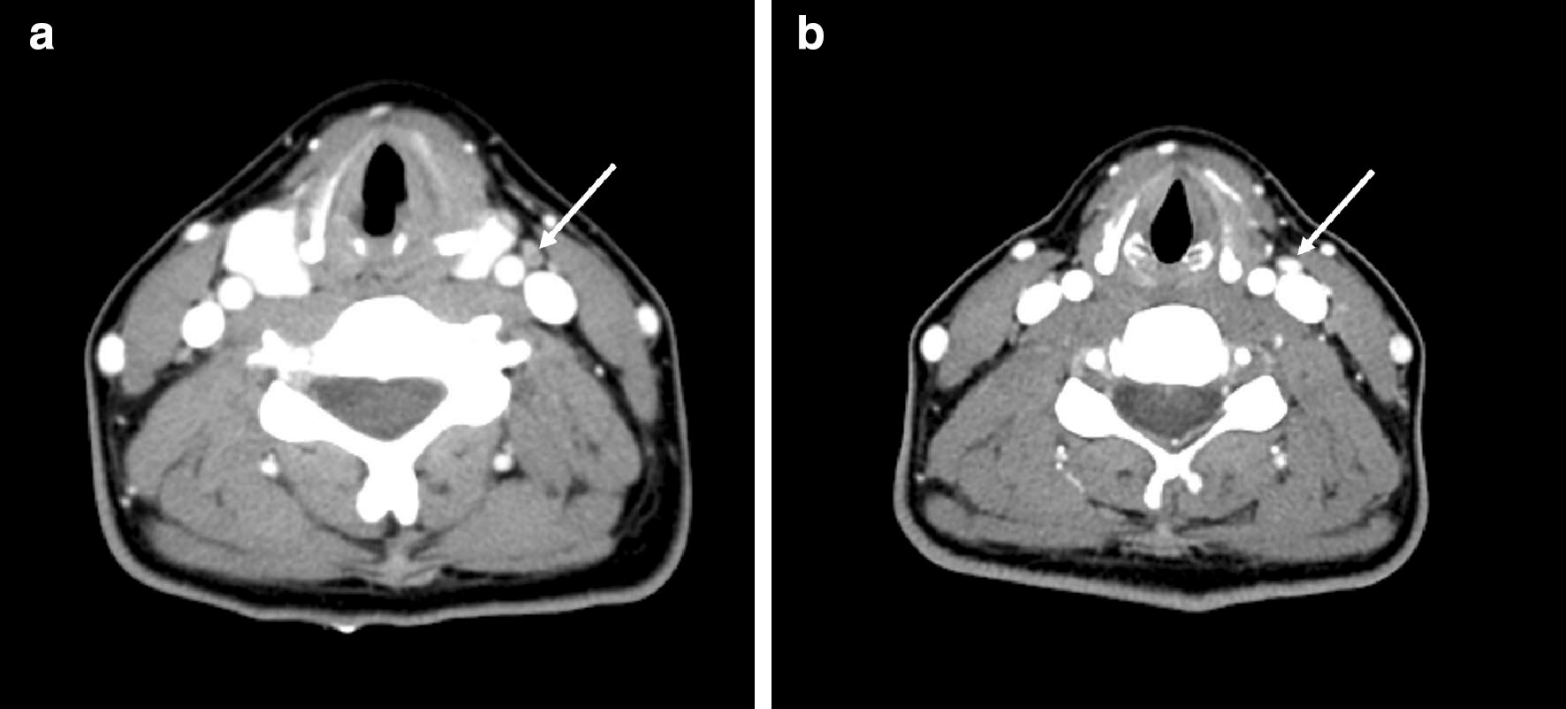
Persistent disease in a 43-year-old woman with bilateral thyroid cancers. (A) Axial CT image with a 60-s scan delay shows a small lymph node at left level III (arrow) without suspicious CT features before initial surgery. She underwent total thyroidectomy with central neck dissection. (B) Axial CT image with a 40-s scan delay shows a lymph node (arrow) with a strong enhancement at the same location before reoperation.
